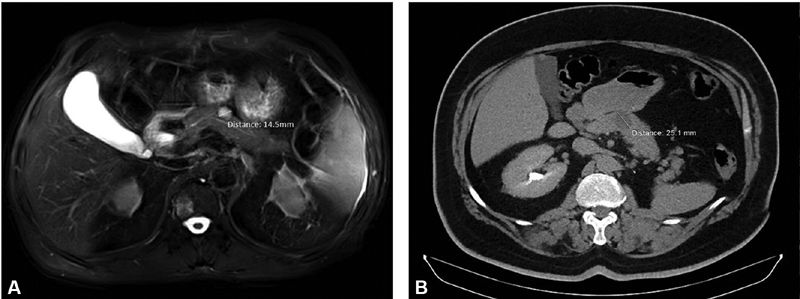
Magnetic resonance imaging showing the pancreatic lesion of (
